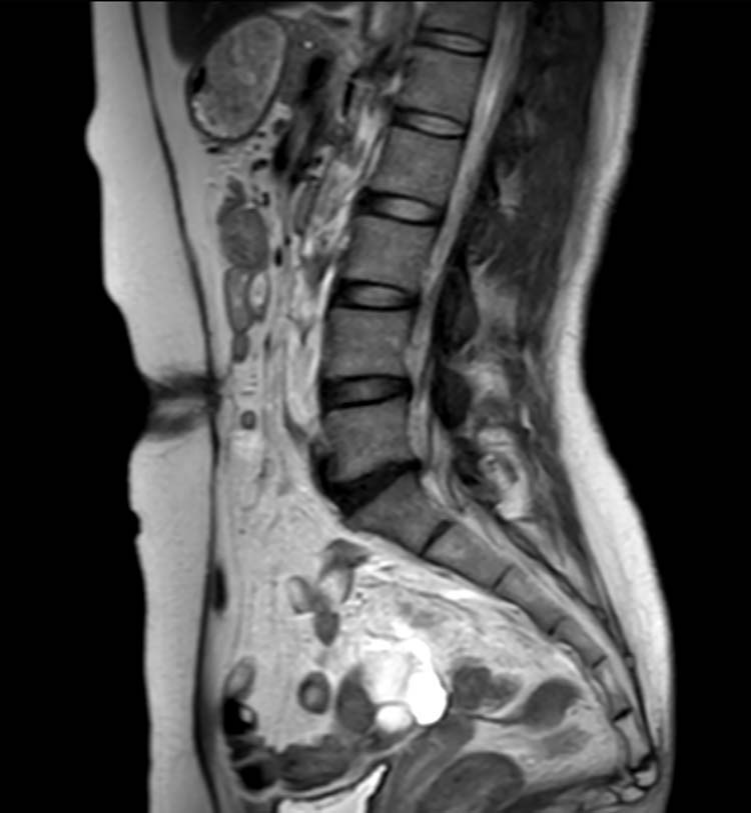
Postoperative MRI. Sagittal view.
